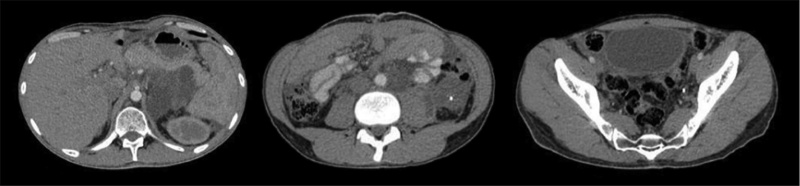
Three slices of the CT scan that was made 6 months after the second surgical resection showing the minimal recurrence of the cyst.
